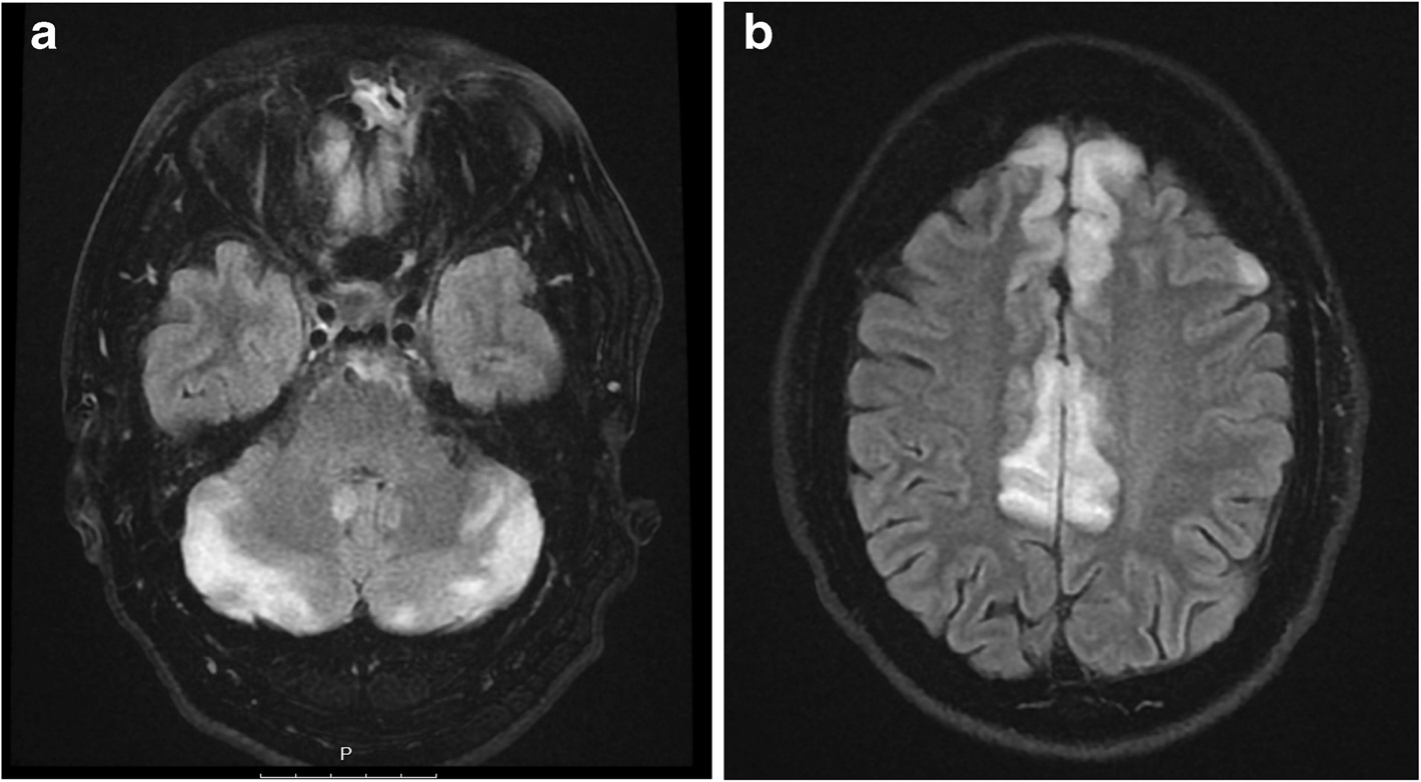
Primary MRI T2 and FLAIR images a and b displaying an increased signal in the cortex throughout the frontal and temporal lobes and in the cerebellum consistent with hypertensive encephalopathy/PRES.
